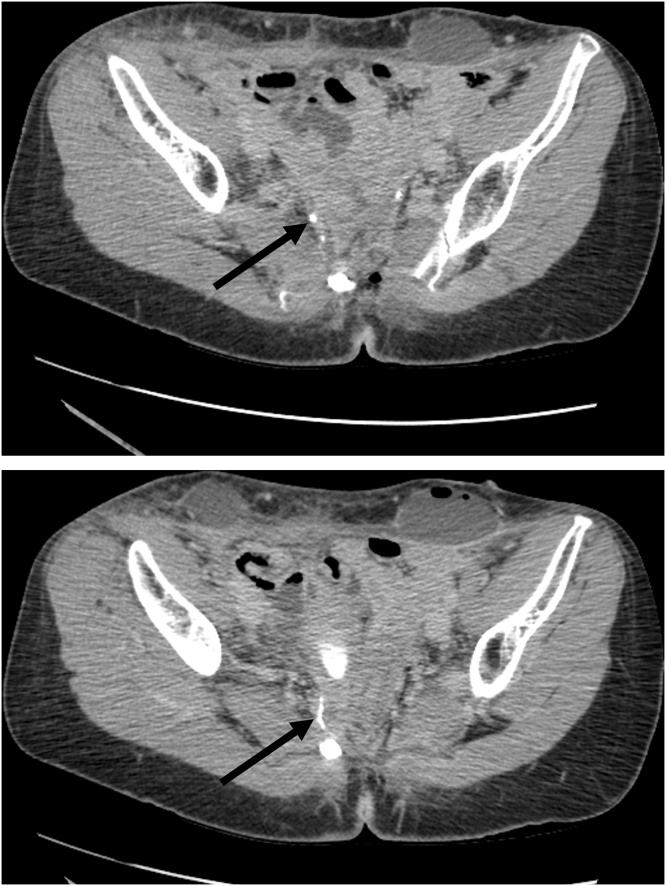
Top. CT with intravenous contrast (07/02/2020). CT slice through the pelvis at the level of S4. The distal right ureter is well demonstrated (indicated by an arrow). Bottom. CT with intravenous contrast. CT slice just beneath the right ureter depicted in Fig. 4, top shows a stream of contrast moving from the right ureter toward the closed suction drain in the presacral space (indicated by an arrow).
